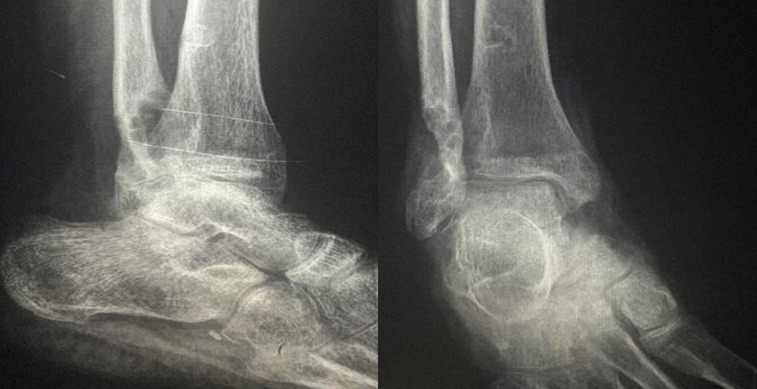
radiographie standard de la cheville gauche: lésion ostéolytique malléolaire externe, à contours flous, sans rupture corticale ni réaction périostée
